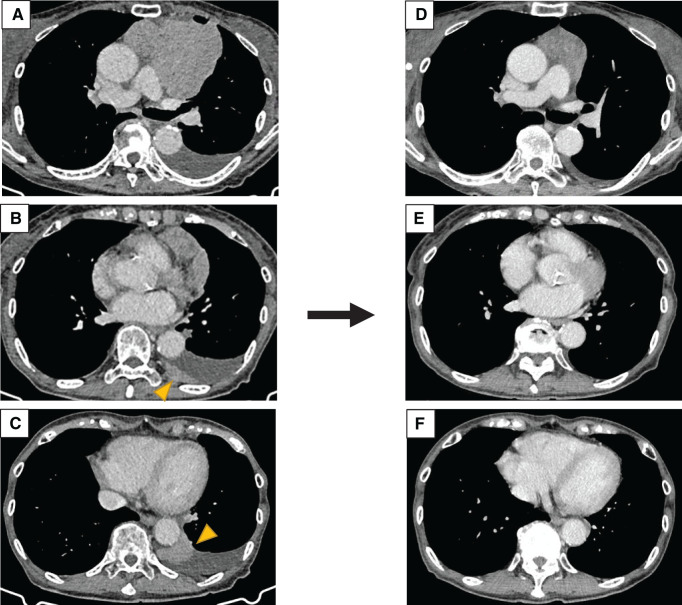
(A) Chest CT before chemotherapy showing a 10-cm diameter mass in the anterior mediastinum. Left pleural effusion due to dissemination was observed. (B) At least 3 sites of pleural dissemination were observed in the left thoracic cavity. This image shows pleural dissemination on the dorsal side of the left thoracic cavity (arrowhead). (C) Disseminated lesions were also observed around the descending aorta (arrowhead). (D) After 2 cycles of CAMP therapy, the primary tumor shrank to 5.1 cm. Pleural dissemination also shrank, and the left pleural effusion decreased. (E, F) The disseminated lesions have almost disappeared. CAMP, cisplatin, doxorubicin, and methylprednisolone
